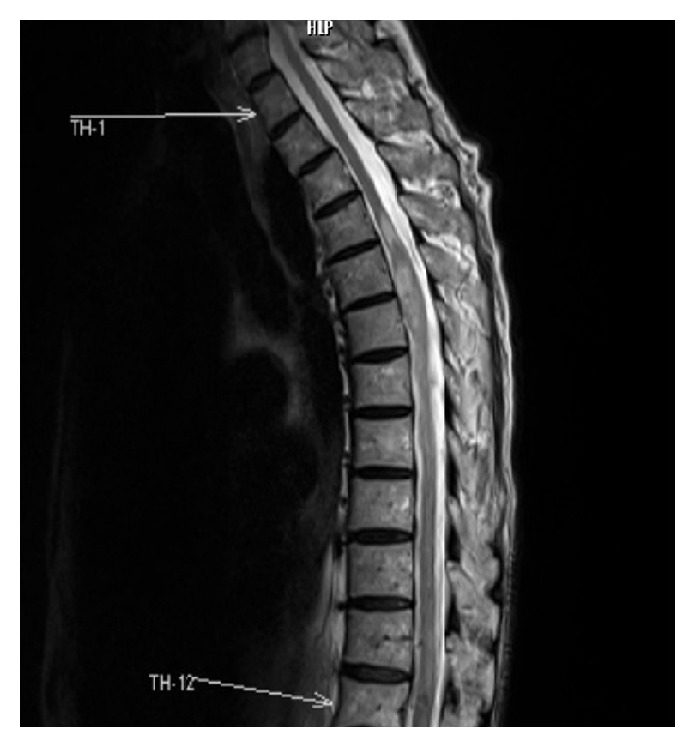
Magnetic resonance imaging (MRI) revealed an intramedullary mass with signal intensity surrounded by a zone of high and low signal on T2 weighted images.
